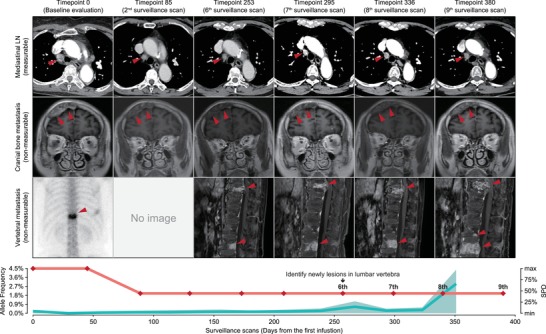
Radiological and serological follow‐ups for P2 with unmeasurable lesions. Upper panels, surveillance CT, MRI scans (with contrast), or ECT showing the change in tumor burden over time in the mediastinal lymph node (LN), cranial bone, and lumbar vertebrae. Red arrowheads indicate the lesion site. Lower panel, clinical course. Red lines, SPD; green line with shadow, mean ctDNA MAF with the range for all detected mutations in ctDNA.
